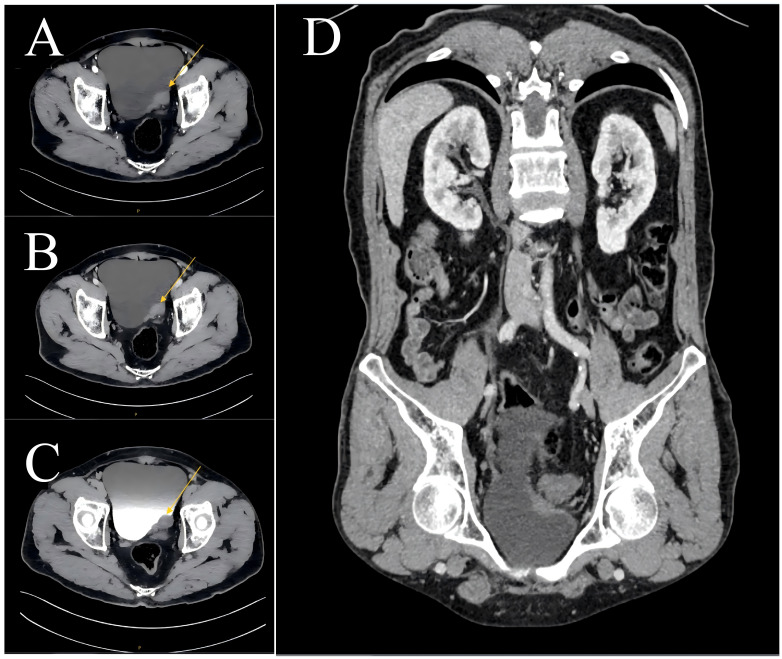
CT images of the male patient before surgical treatment. An irregular mass shadow (yellow arrow) measuring approximately 4 cm × 2 cm is visible at the left ureteric orifice, with adjacent bladder wall thickening and enhancement. (A) Arterial phase in the transverse position; (B) Venous phase in the transverse position; (C) Delayed phase in the transverse position; (D) Coronal CT manifestation.
